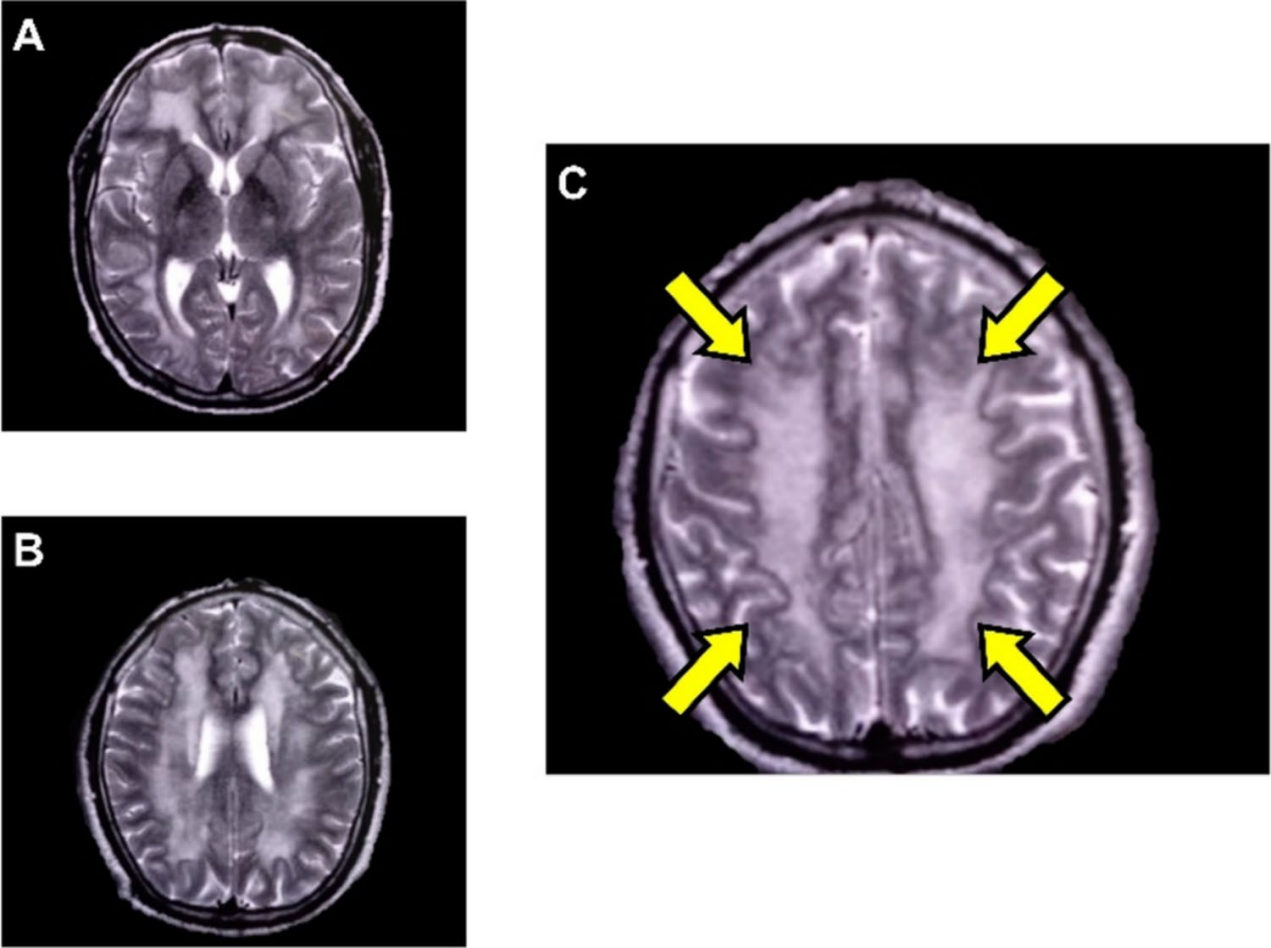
MRI showing extensive ischemic-type lesions in the white matter of the semi-oval centers A) Deep centers, B) High centers & C) Medial centers, evidenced by hyperintensity on diffusion, suggesting leukoencephalopathy
